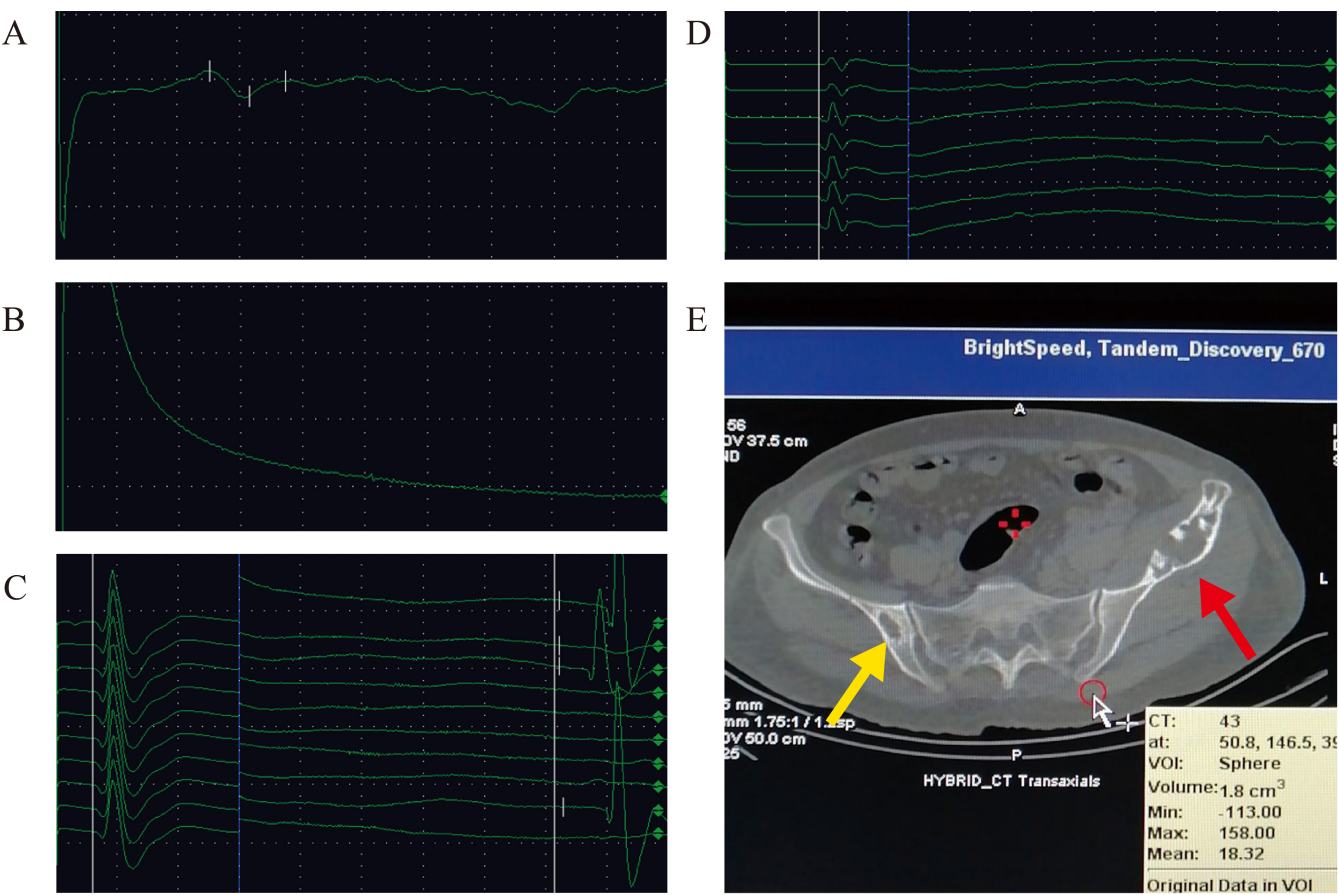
Electromyography examination and sacroiliac joint CT scan. (A) The sensory conduction velocity of the left ulnar nerve exhibited a marked reduction. (B) The sensory conduction velocity testing of the right peroneal nerve failed to evoke positive waveforms. The F-waves of the left ulnar nerve (C) and left median nerve (D) were not elicitable (E). CT scan of the sacroiliac joint (refer to annotations in the lower right corner): limited linear high-density shadows (yellow arrow) are observed on the right sacroiliac joint iliac bone surface, with irregular low-density shadows suggesting bone destruction and a “doughnut-shaped” distribution of contrast agent, accompanied by local sclerosis. On the left iliac wing, expansive osteolytic lesions are evident (red arrow), with adjacent thinning of the cortical bone. Spotty calcifications are noted within, and there is no apparent soft tissue mass in the surrounding area. The tissue biopsy site is also indicated by the red arrow, which points to the soft tissue mass on the left side.
